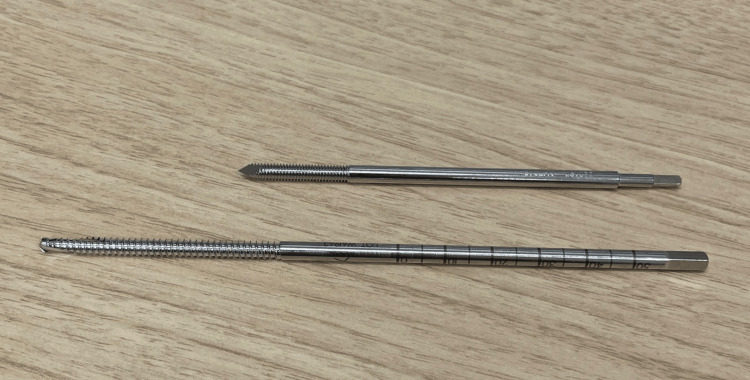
The upper pin represents the pin for CT navigation, and the lower pin represents the pin for the Mako. The pin for the Mako has a longer threaded section and a larger threaded interval than the one for CT navigation.
